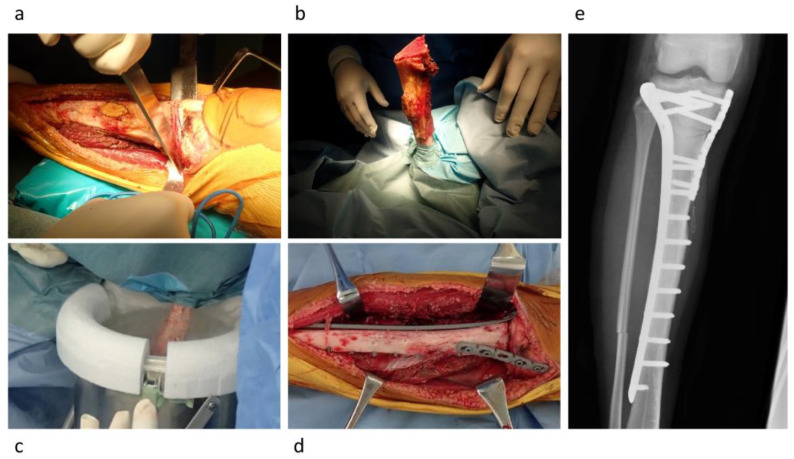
Pedicle freezing procedure. (a) Osteotomy was performed in the proximal site with surgical margin. (b) The tumor-bearing bone was isolated by surgical sheets. (c) The tumor-bearing bone was frozen in liquid nitrogen by rotation of the affected limb. (d) After the freezing, the tumor-bearing frozen bone was used for reconstruction. (e) Postoperative X-ray.
